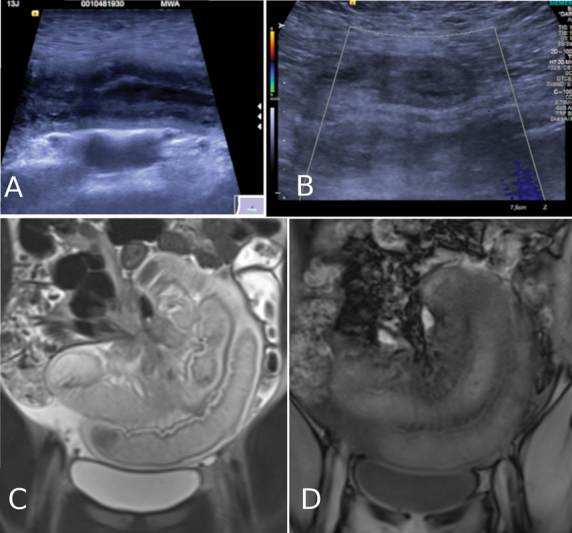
Notable on ultrasound is the appearance of dilated, thickened bowel loops (
